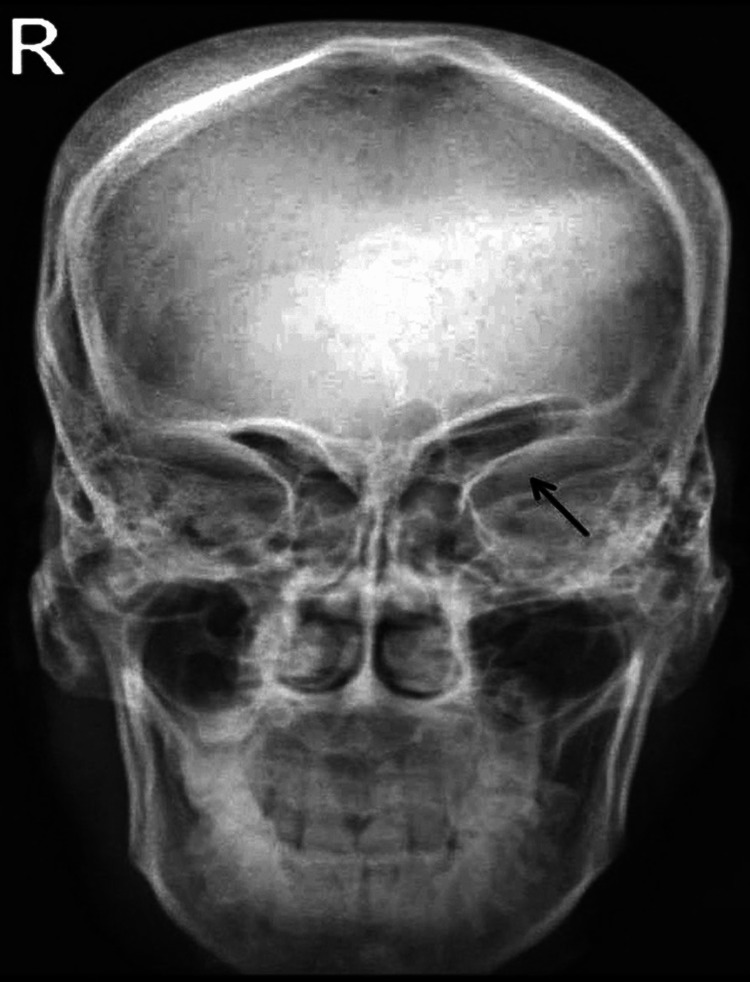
X-ray of the skull reveals hyperpneumatization of the left sinus (black arrow)
